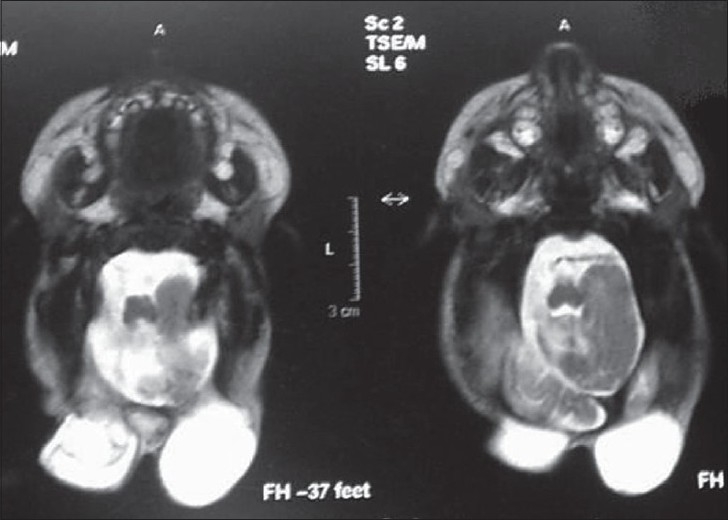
MRI image showing two separate sacs without any neural tissue in them. Note the normal piece of bone in between the two sacs (arrow)
